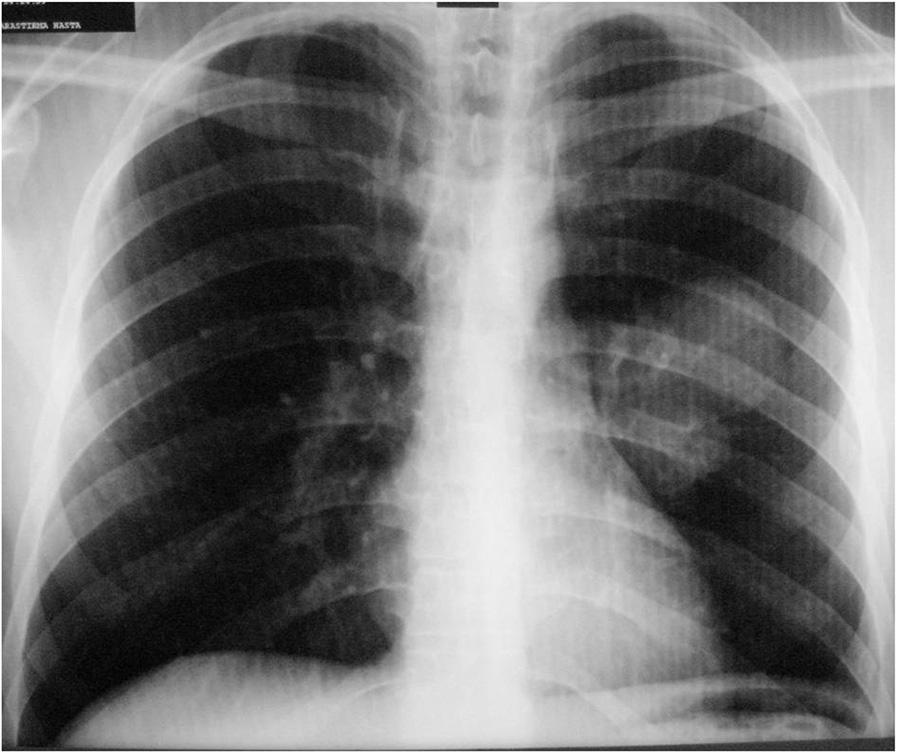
Chest radiography is showing the well-shaped, round hilar opacities on left hemithorax.
